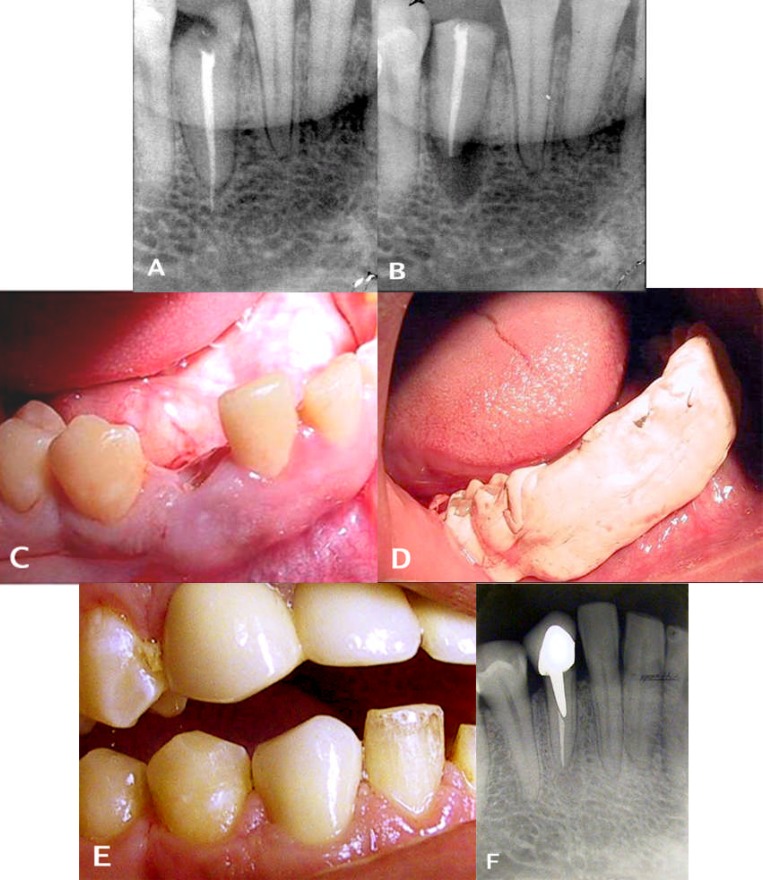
A. Preoperative radiograph appearance of a crown- root fracture on tooth 27.
